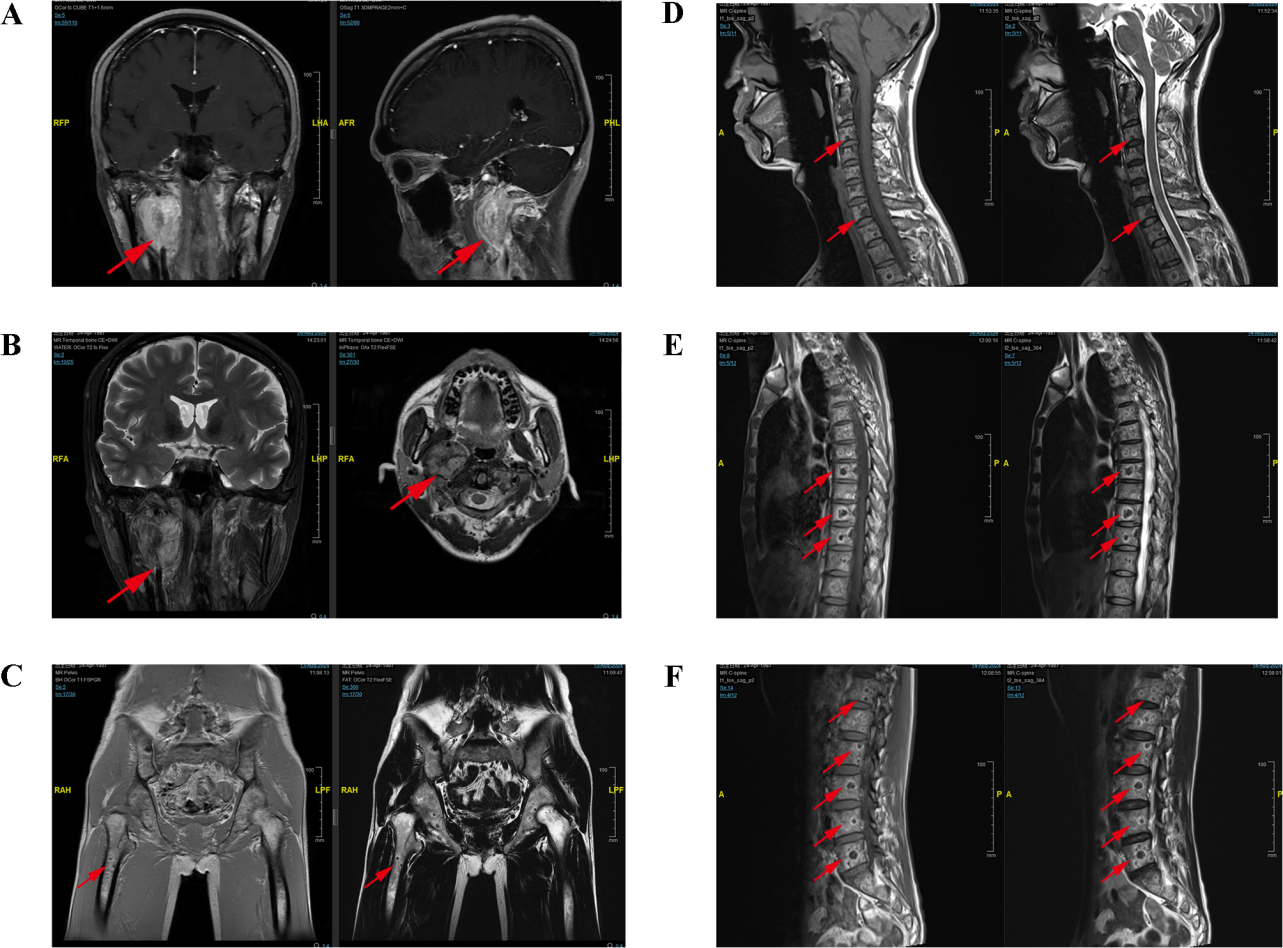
The MRI examination images of the patient. The MRI of the head (A, B) demonstrated an oval-shaped space-occupying lesion between the internal carotid artery and vein in the right parapharyngeal space, exhibiting uneven signal intensity and heterogeneous enhancement, with a larger section measured approximately 38mm×29mm×43mm. The MRI of the pelvis (C), cervical vertebra (D), thoracic vertebra (E) and lumbar vertebra (F) indicated the presence of multiple flaky and quasi-circular abnormal signal shadows in the pelvic bones and vertebral bodies. The MRI showed multiple patchy, quasi-circular abnormal signal shadows, with low signal intensity on T1WI and T2WI accompanied by surrounding ring-shaped high signal intensity shadows and slightly high signal intensity on fat-suppressed T2WI accompanied by peripheral annular low signal intensity shadows, which showed special ring signs.
